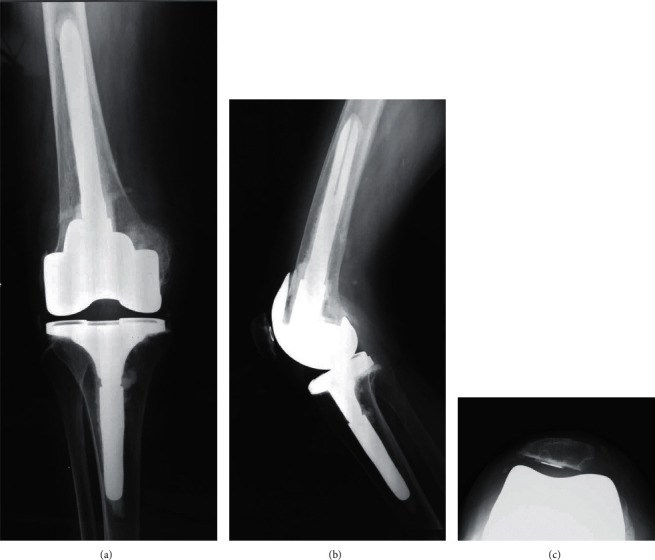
Postoperative radiographs showing total knee arthroplasty using the semiconstrained lesion prosthesis (Smith & Nephew). (a) AP view, (b) lateral view, and (c) axial view.
